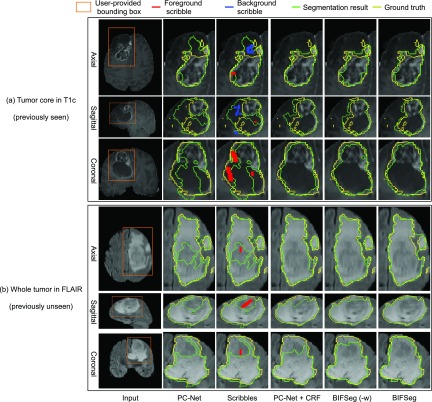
Visual comparison of PC-Net and three supervised refinement methods with scribbles for 3D brain tumor segmentation. The refinement methods use the same initial segmentation and set of scribbles. (a) Tumor core in T1c (previously seen). (b) Whole tumor in FLAIR (previously unseen).
